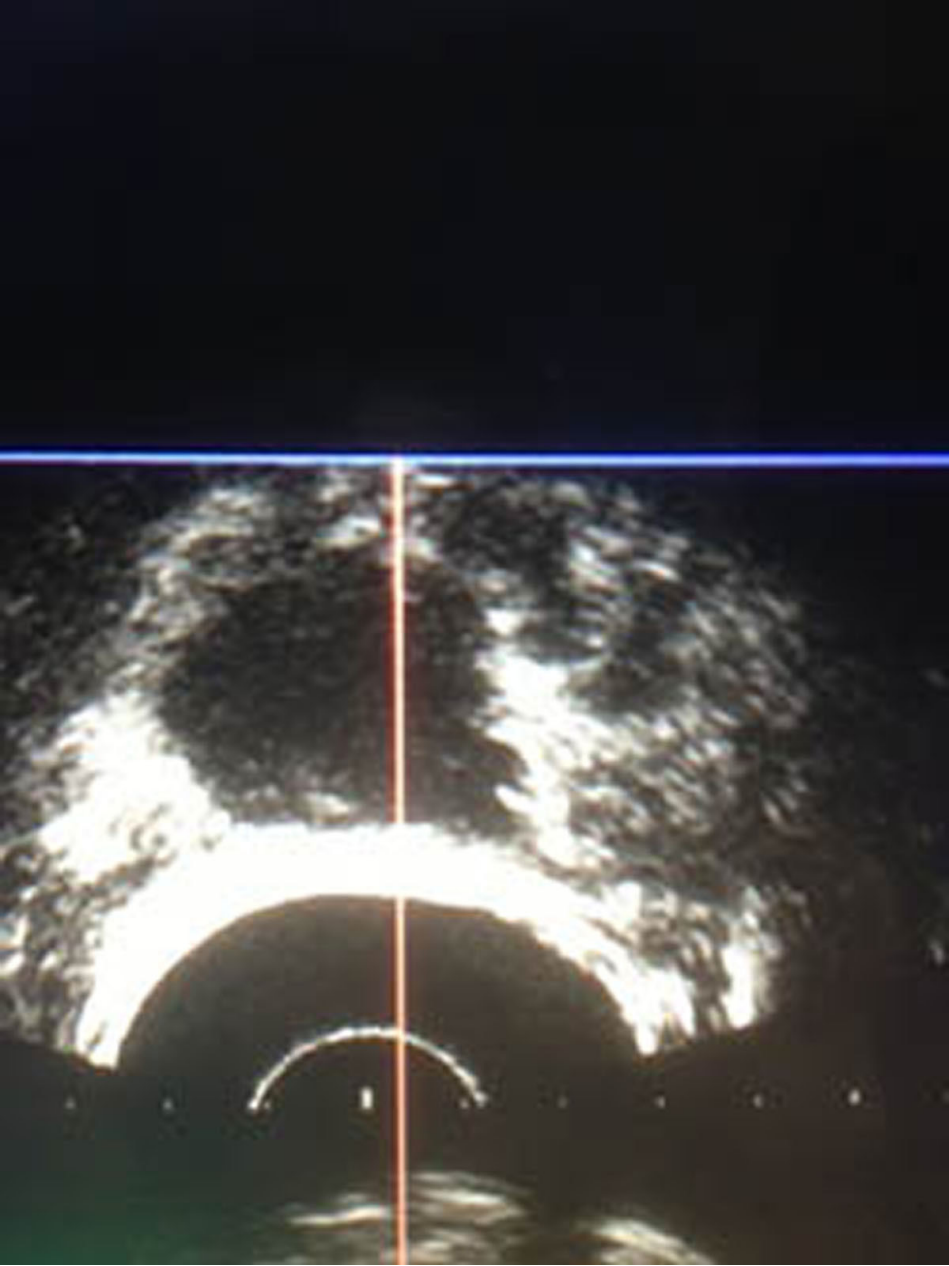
Contrast-enhanced Ultrasound showing the de-vascularized areas.
